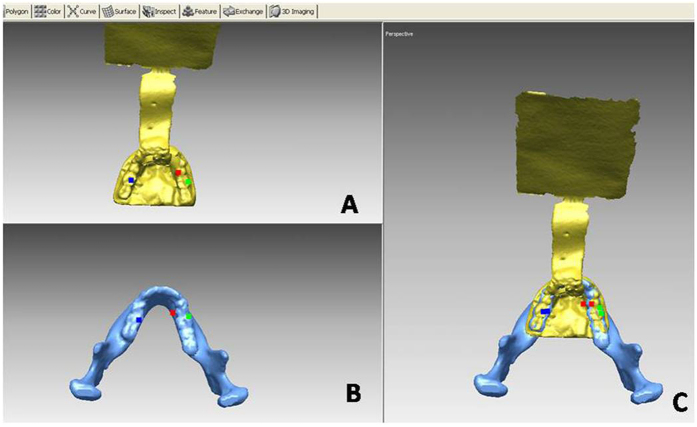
Virtual integration process. The virtual images of the dental cast and mandible were integrated using 3 designated points. (A) The scanned 3D model of the occlusal splint and tracking marker; (B) the model of the mandible from CT data using volume rendering; (C) the well-prepared virtual image for performance in an AR system.
